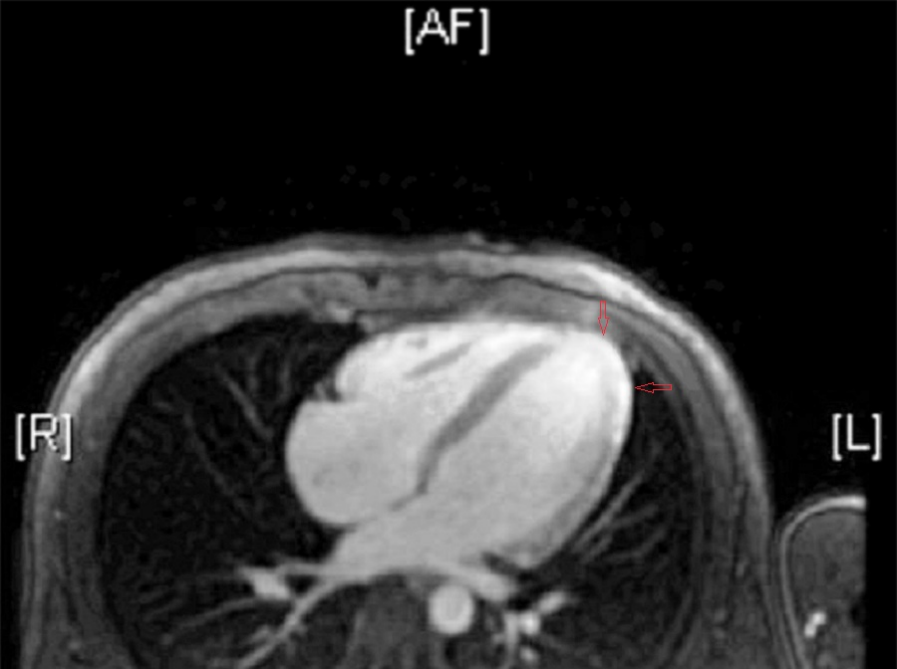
Cardiac MRI Direct axial image of cardiac MRI showing sub-epicardial enhancement in basal to apical anterolateral, inferiolateral and anteroseptal wall. MRI - magnetic resonance imaging.
